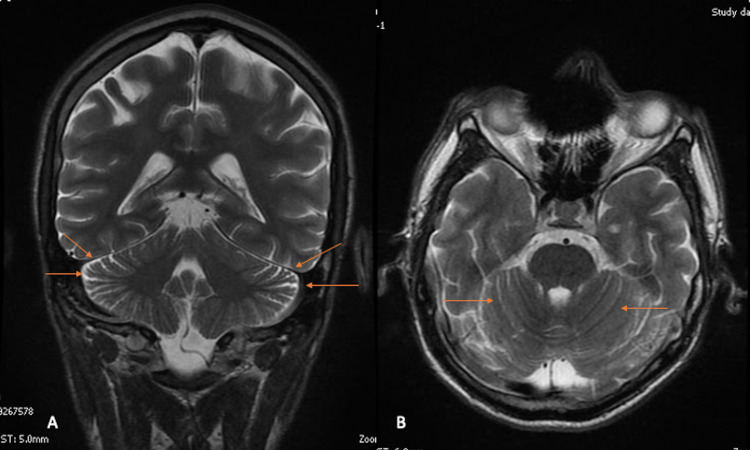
T2-weighted brain MRI in coronal view (A) and axial view (B) showing (arrows) a slight accentuation of cerebellar folia in the context of a patient with Capgras syndrome. MRI: magnetic resonance imaging
